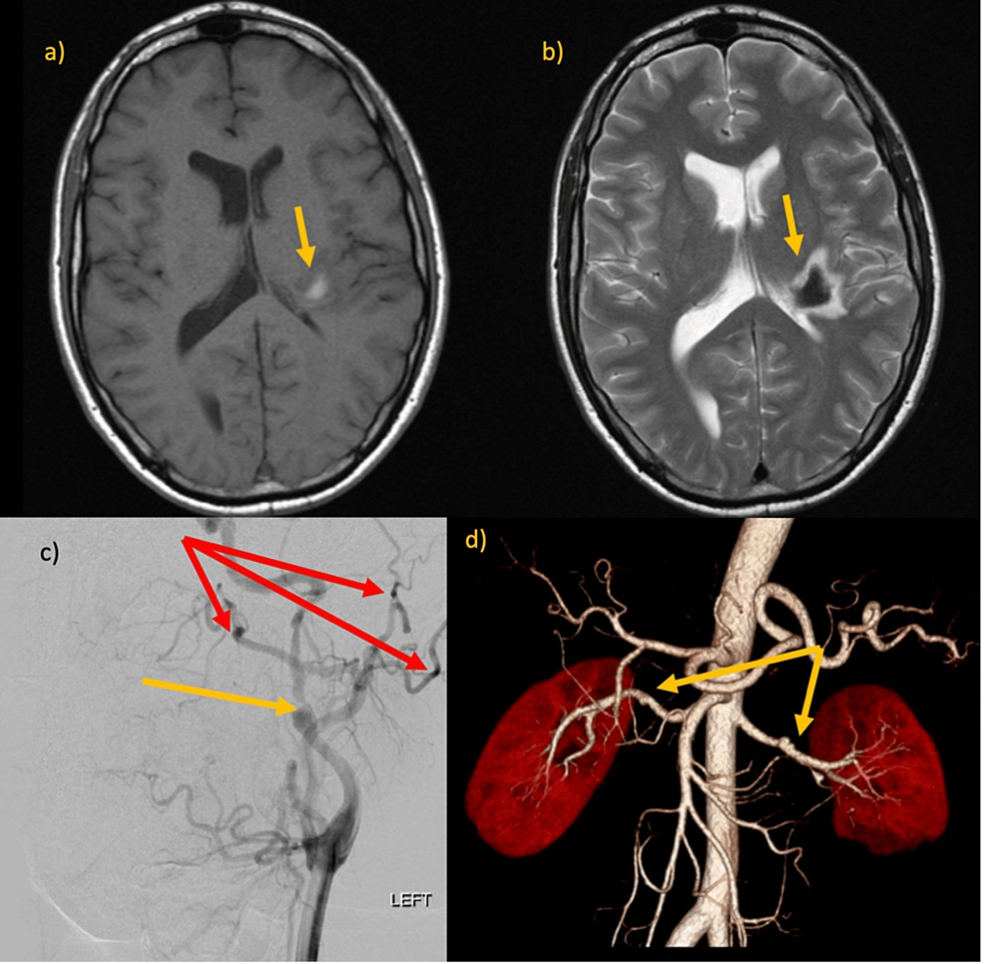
MRI brain T1 (a) and T2 (b) demonstrating left corona radiata acute haemorrhage (highlighted by arrows). (c) DSA demonstrating left ICA high grade stenosis (highlighted by yellow arrow) and left ECA focal irregularities (highlighted by red arrows). (d) 3D reconstruction of CT angiogram demonstrating bilateral renal artery irregularities and normal mesenteric visceral arteries (highlighted by arrows).
